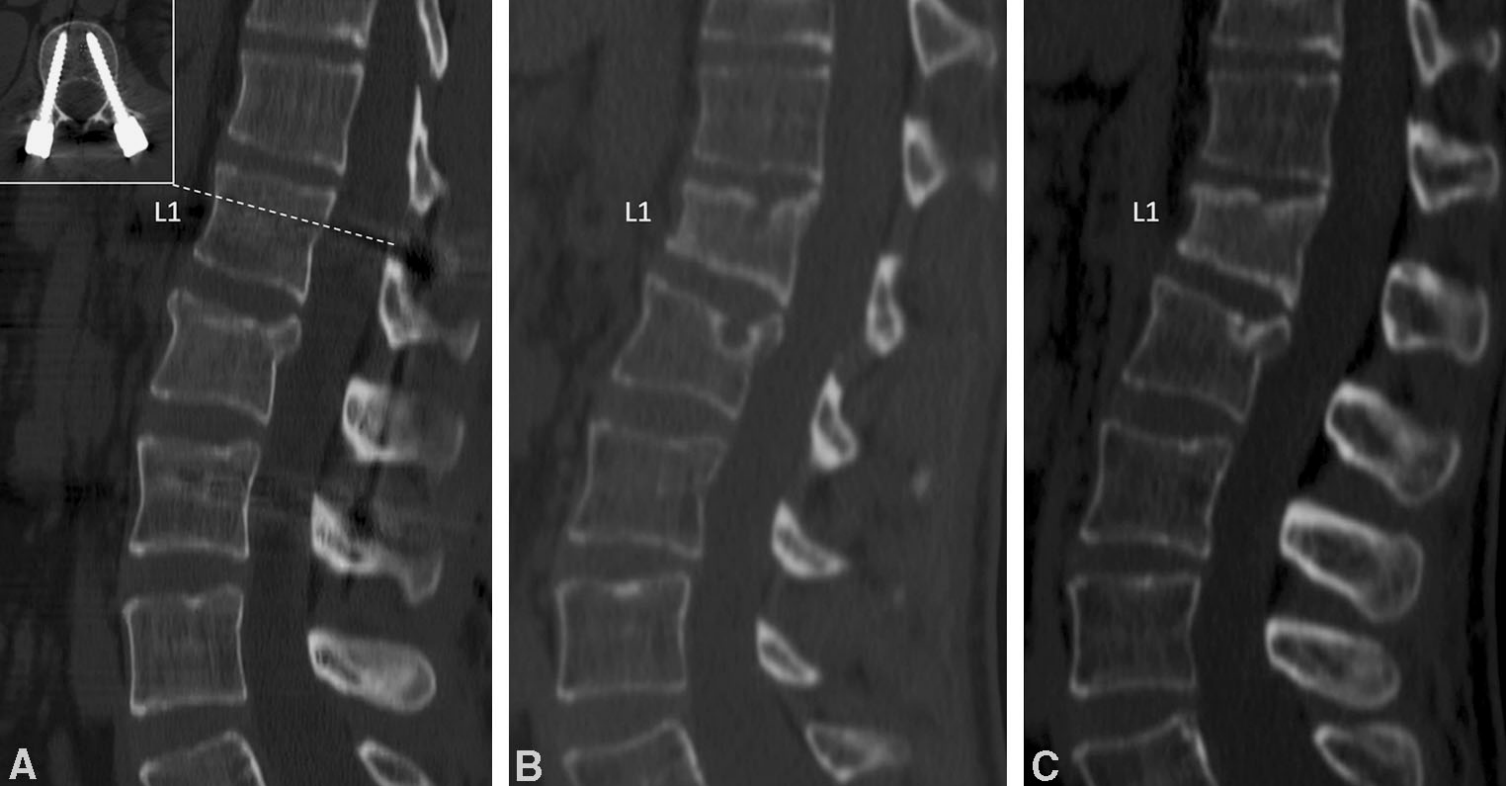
a Postoperative CT scan showed a good reduction of the fracture with correct positioning of pedicle screw in L1 (in the *box*). b One month after implant removal, a CT scan showed an acute wedge fracture on L1. c Fracture healing after conservative treatment with 3-point brace for 3 months
